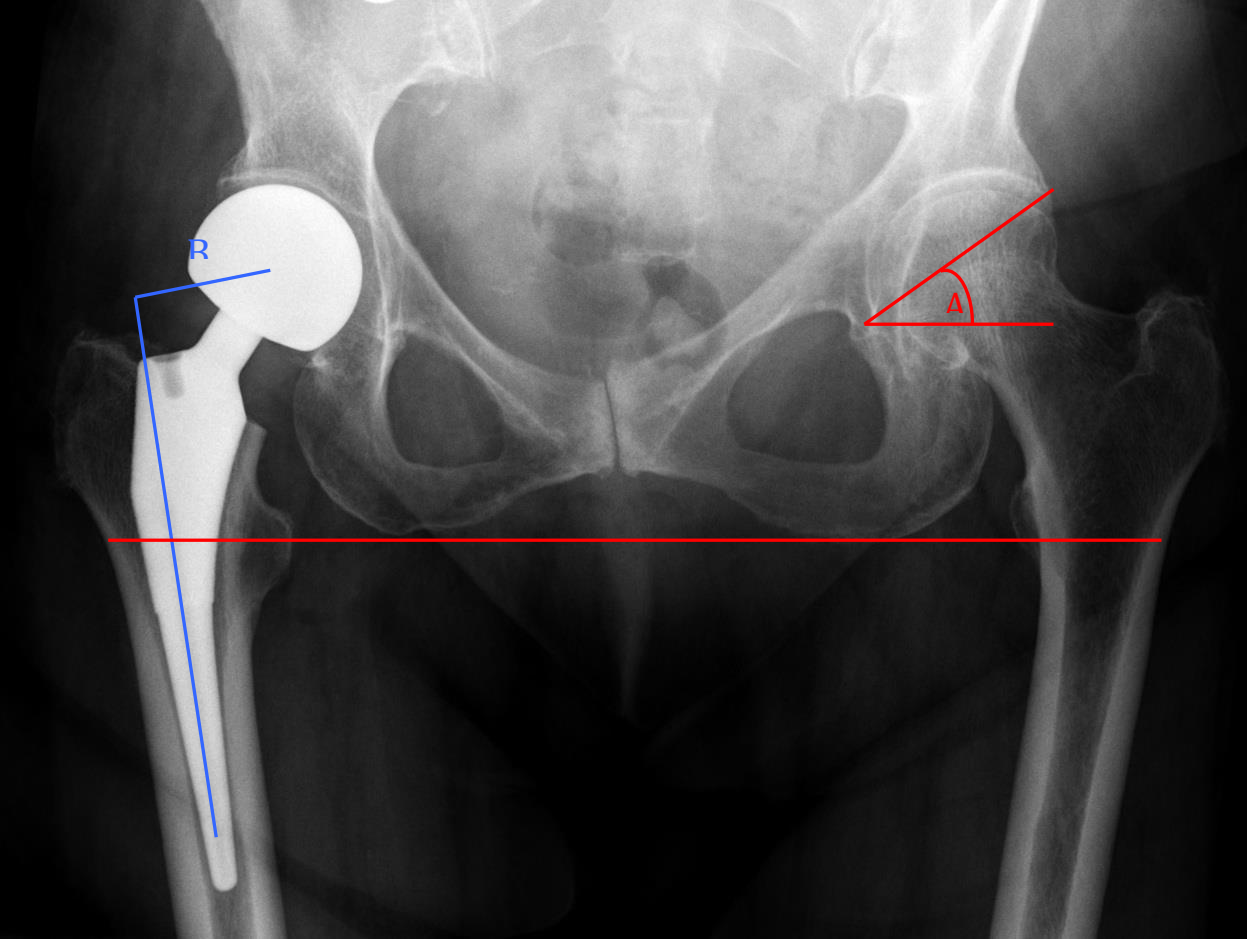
Radiograph of an uncemented hemiarthroplasty with a unipolar head showing the acetabular index (A - red) and femoral neck offset (B - blue).
